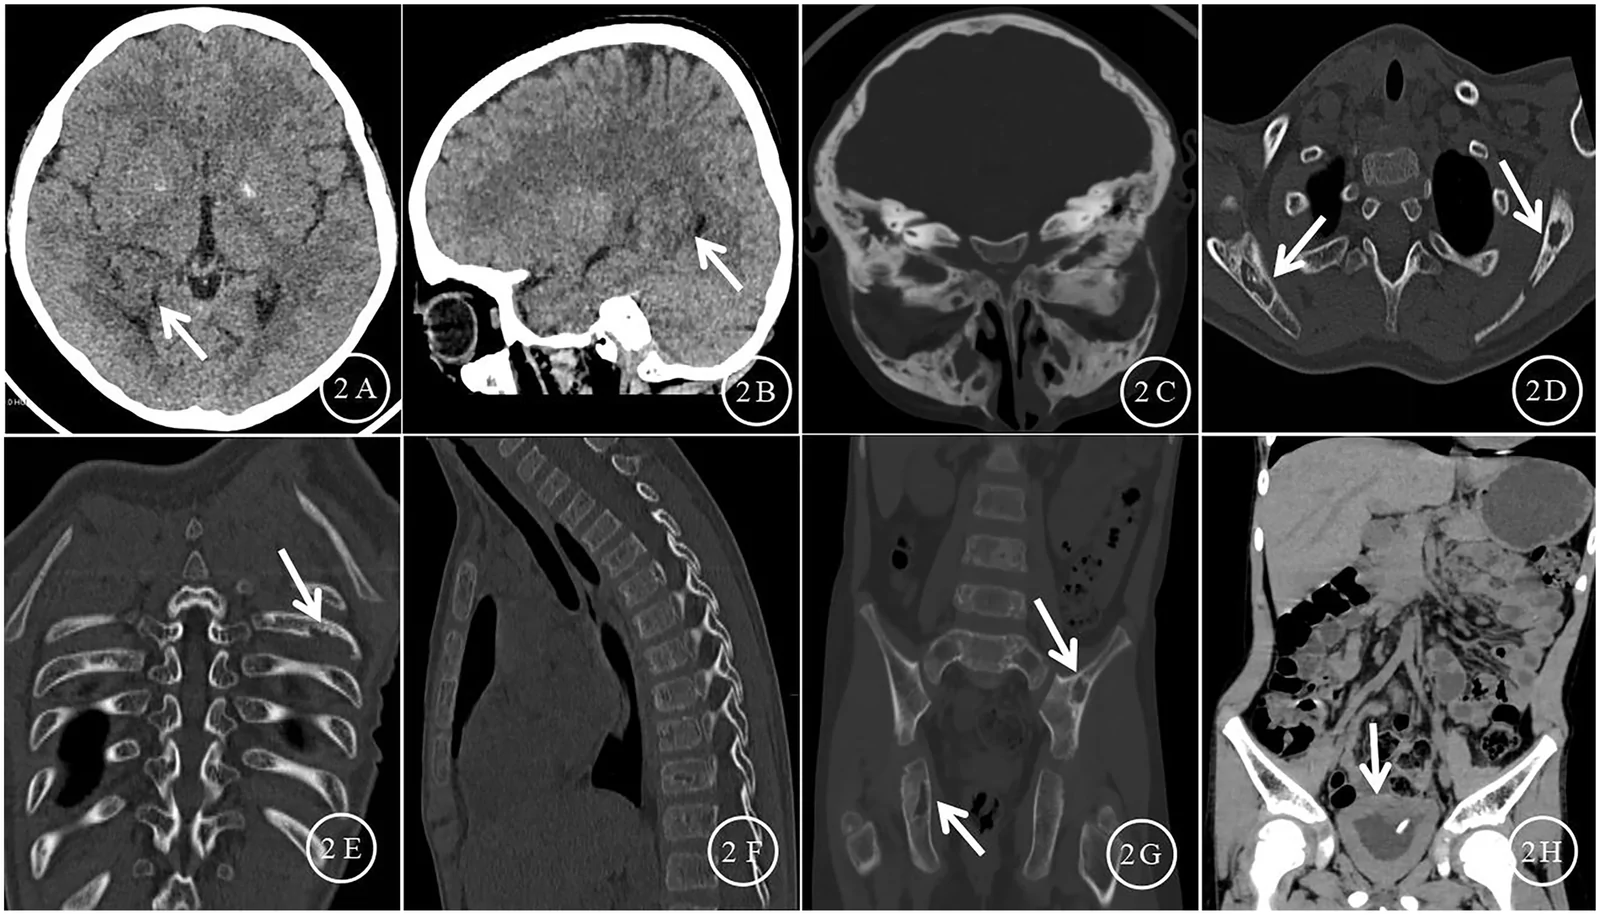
CT findings of ECD. (A,B) Axial and sagittal CT: a knotted, patchy, mixed-density lesion in the right lateral ventricle trigone (white arrow). (C–G) Extensive hyperdensity of the facial bones, skull base, and calvarium, with irregular contours; heterogeneous bone density of the scapulae, sternum, ribs, vertebrae, and pelvis (white arrows). (H) Diffuse bladder wall thickening (≈1.6 cm, white arrow).
